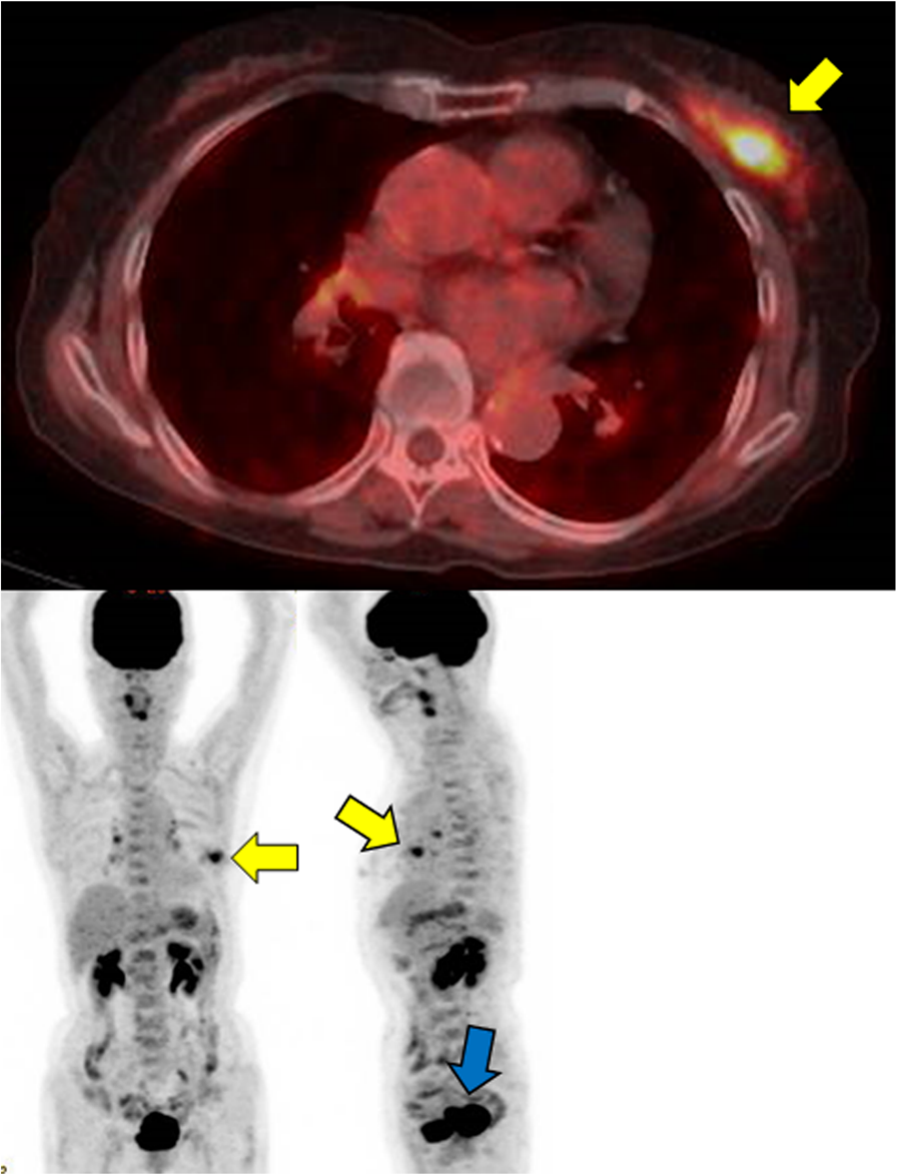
a, b Whole body fluorodeoxyglucose (FDG) positron emission tomography-computed tomography (PET-CT) images. PET-CT shows a mass in the left breast with a maximum standardized uptake value of 4.4 (*yellow arrows*). b It also shows a nodular lesion in the cervix uteri, with high FDG uptake (*blue arrow*)
